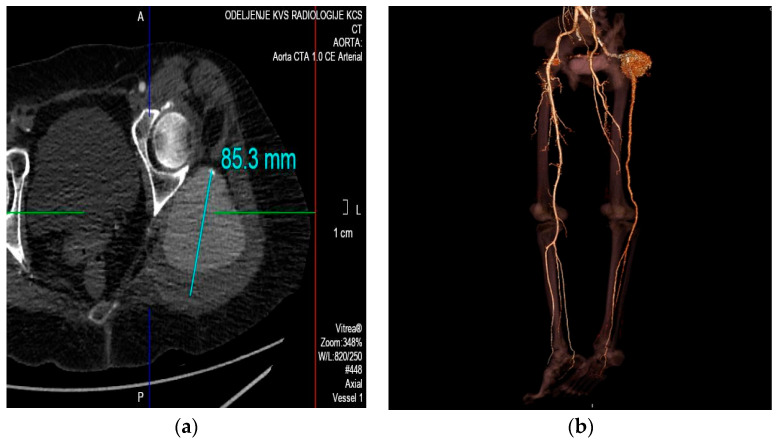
Computed tomographic arteriogram. (a) Axial cross section showed a pseudoaneurysm that measured 85 mm in diameter, with the parietal thrombus on posterior wall. (b) VR reconstruction revealed a large pseudoaneurysm of the left persistent sciatic artery, as well as the usual anatomical presentation of the right iliac and femoral arteries.
